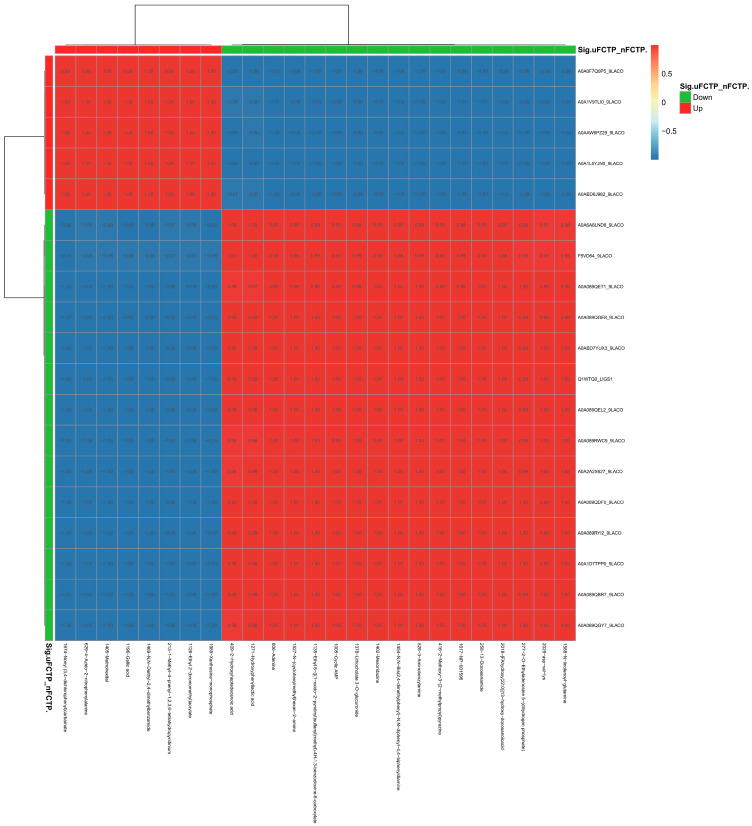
Top 30 positive correlations between differential metabolites and proteins in the uFCTP versus nFCTP comparison. nFCTP: native FCTP; uFCTP: ultrasonically degraded FCTP.
